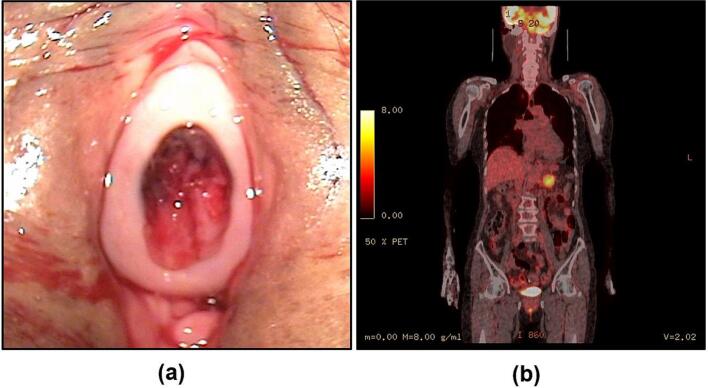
(a) Urethral caruncle: a blood‑tinged sessile polypoid mass located posterior aspect of urethral meatus. The mass was friable and clearly the source of bleeding. (b) PET-CT scan previously reported no specific comment on the urethral region: Retrospective review after the pathologic confirmative diagnosis revealed suspicious high [18]FDG uptake in the region.
